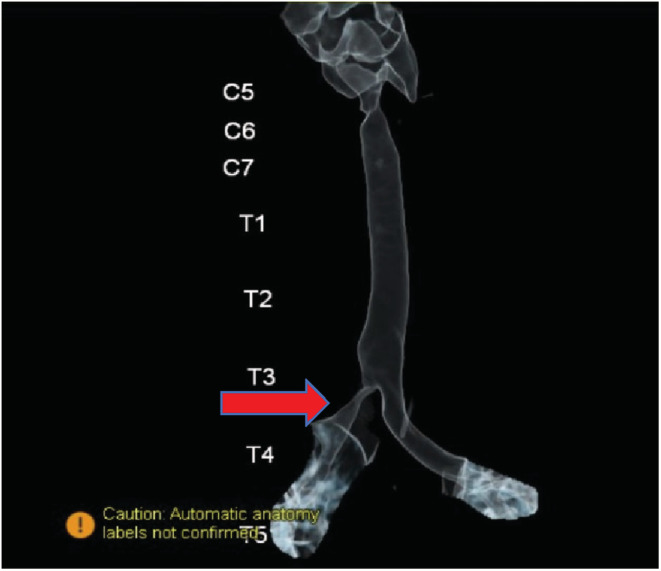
Partial narrowing of right main bronchus at the levels of third thoracic in the 3‐dimensional chest CT scan (virtual bronchoscopy)
